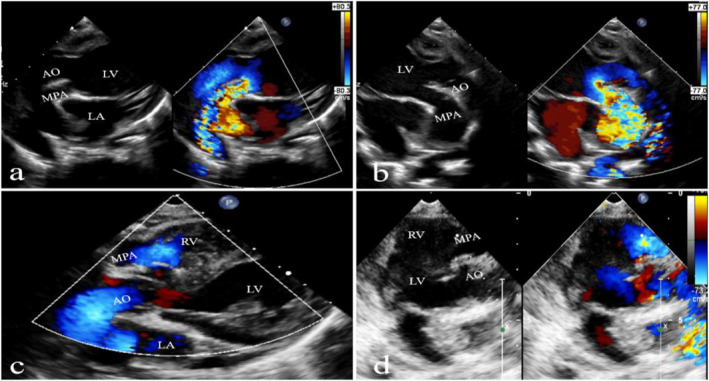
Echocardiographic images in a case of levocardia with the right ventricular loop are presented. Preoperative nonstandard parasternal five chamber view (a) and nonstandard parasternal left ventricular long axis view (b) show that aortic widening straddles the interventricular septum, with the aorta mostly originating from the left ventricle and pulmonary artery stenosis, also arising from the left ventricle. Postoperative images (c and d) show that the aorta connects to the left ventricle and the pulmonary artery connects to the right ventricle, which correspond to the preoperative views in panels (a) and (b), respectively. AO, aorta; LA, left atrium; LV, left ventricle; MPA, main pulmonary artery; RA, right atrium; and RV, right ventricle.
